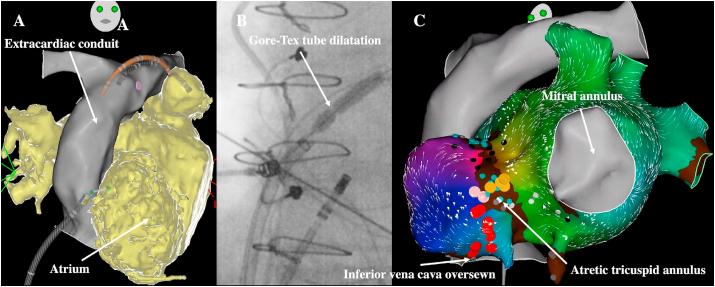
Atrial flutter catheter ablation in a patient with extracardiac Fontan surgery. A: 3D anatomical reconstruction of the extracardiac conduit (gray) and merging with the computed tomography (CT) scan (right atrium in yellow) to locate the optimal puncture site (right anterior oblique view). A reference decapolar catheter is inserted in the left pulmonary artery B: *Trans*-Gore-Tex tube puncture with balloon expansion to introduce the sheath C: Biatrial activation mapping using high-density catheter (Pentaray, Biosense) revealing a macro IART around the atretic tricuspid annulus with linear ablation performed between the atretic tricuspid annulus and the scar where the inferior vena cava was oversewn (left anterior oblique view). Yellow dots denote His signal, red dots denote RF applications.
